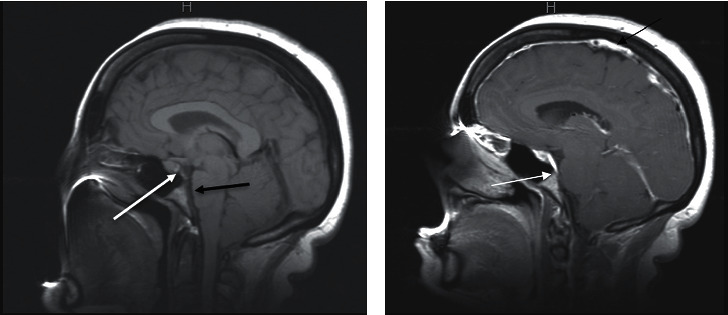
(a) Sagittal T1-weighted MRI image demonstrating the sagging brain and downward displacement of the cerebellum, pituitary enlargement, closure of the midbrain-pons angle (white arrow), and flattening of the pons (black arrow). (b) Postcontrast sagittal T1 image demonstrating diffuse pachymeningeal enhancement, engorgement of dural venous sinuses (black arrow), and obliteration of the prepontine cistern (white arrow).
